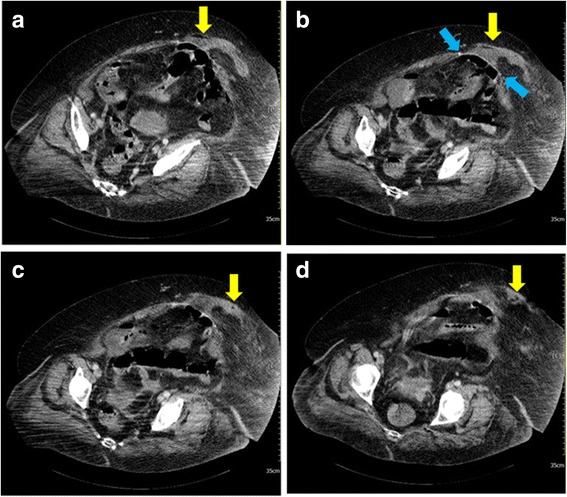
Computed tomography scan, contrast-enhanced transverse view, cranial (a) to caudal (d). Series of subcutaneous fluid accumulation (*yellow arrows*) extending from the mesh (between *blue arrows*) to the superficial incision wound
